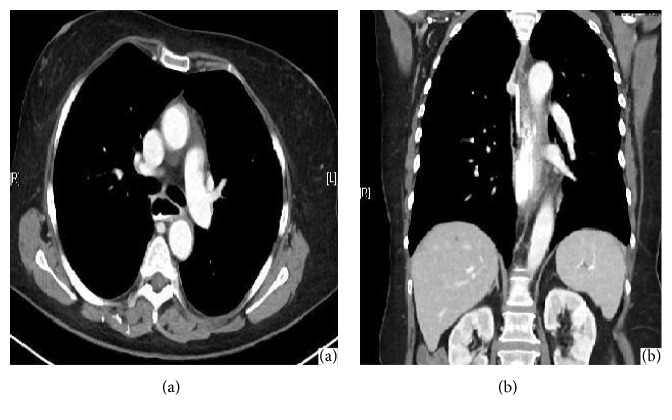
Follow-up CT showing resolving haematoma ((a) sagital and (b) coronal view).
